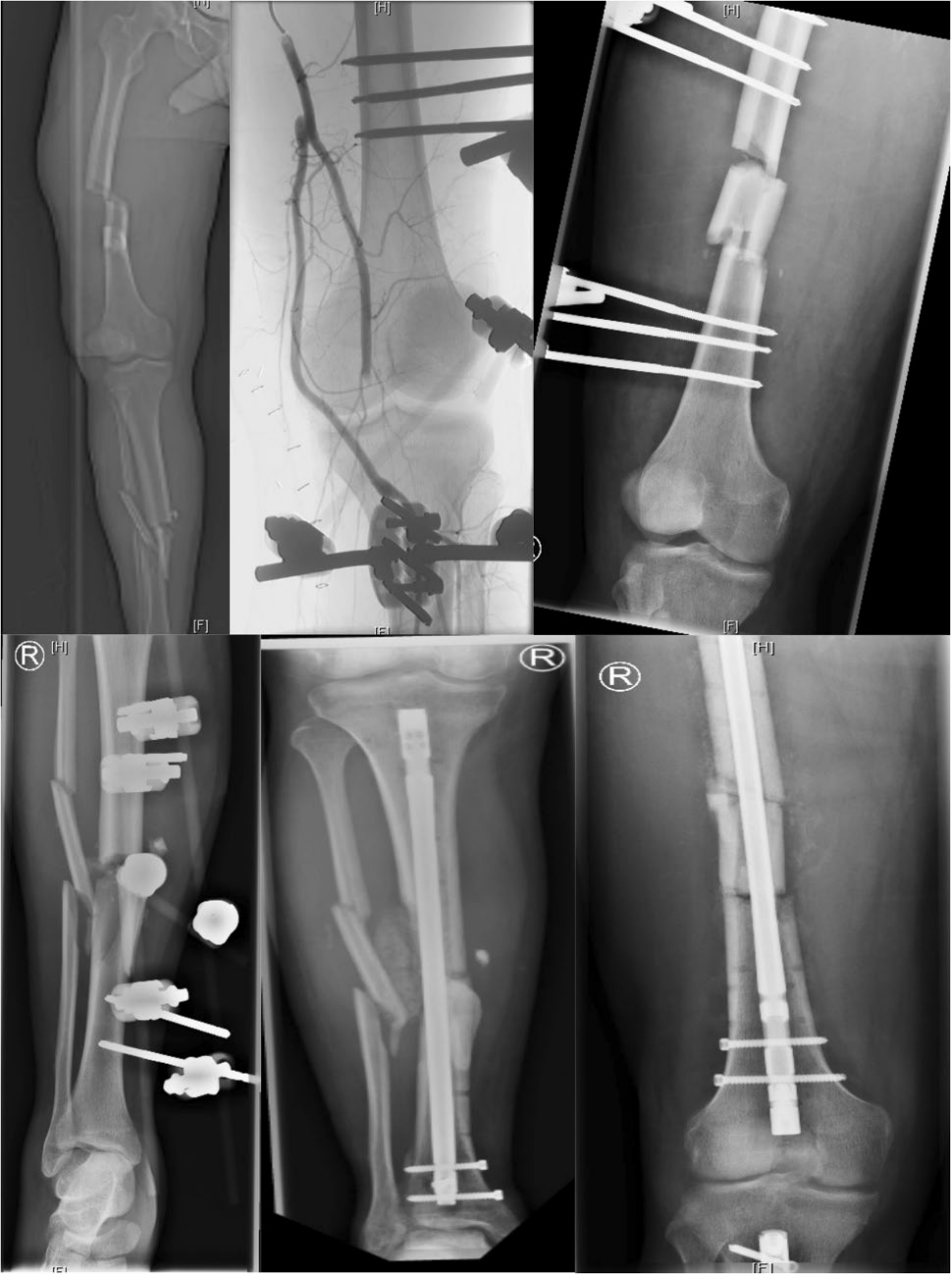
Anterior–posterior radiographs and angiography of a 32-year-old patient with a complete knee dislocation in a motorcycle accident. The figure shows the initial CT-scout of the limb, the ruptured popliteal artery as well as the leg after treatment in stages with vein graft, external protective fixation, and intramedullary nails prior to ligament reconstruction
